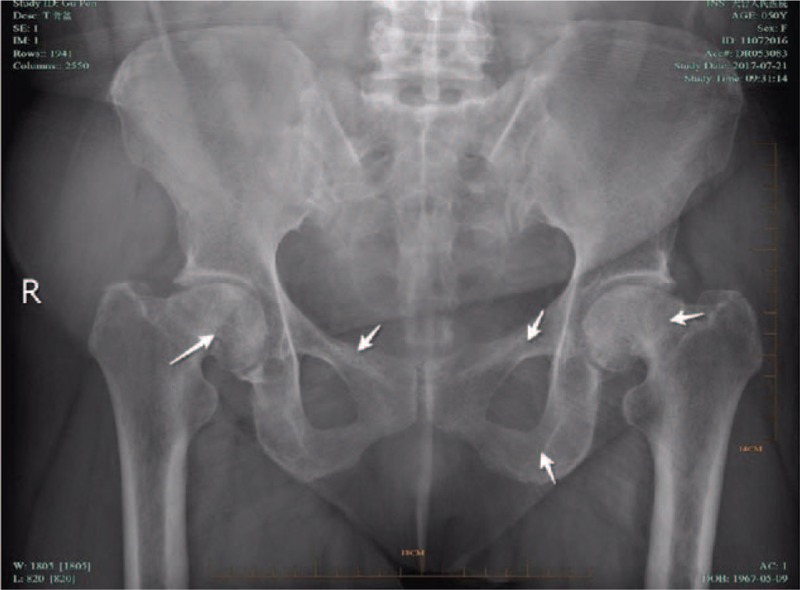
Postoperative pelvic X-ray at 7 months showed blurred fracture lines for both bilateral femoral neck and bilateral pubic fractures (indicated by white arrow).
